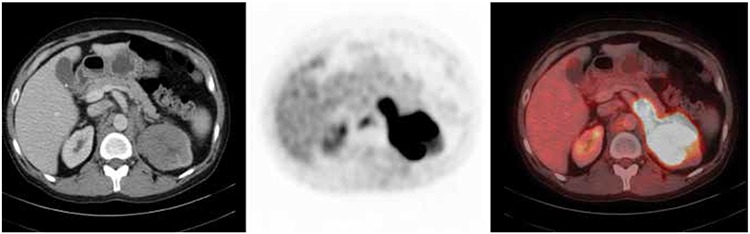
A 43-year-old-male patient with a diagnosis of metastatic neuroendocrine tumor on cervical node biopsy. Axial positron emission tomography-computed tomography and fusion images demonstrate hypermetabolic left renal soft tissue mass (SUVmax: 13.9). The biopsy of this renal mass revealed a neuroendocrine tumor
